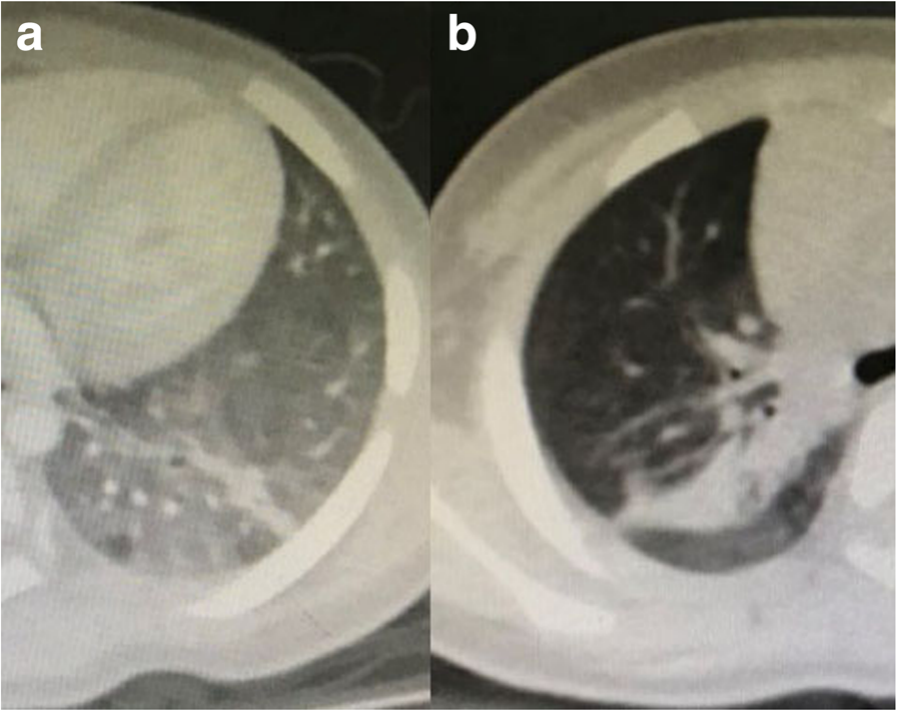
Chest CT. Increased patch density in the left lower lobe (a) and the right upper lobe (b) of the lung
